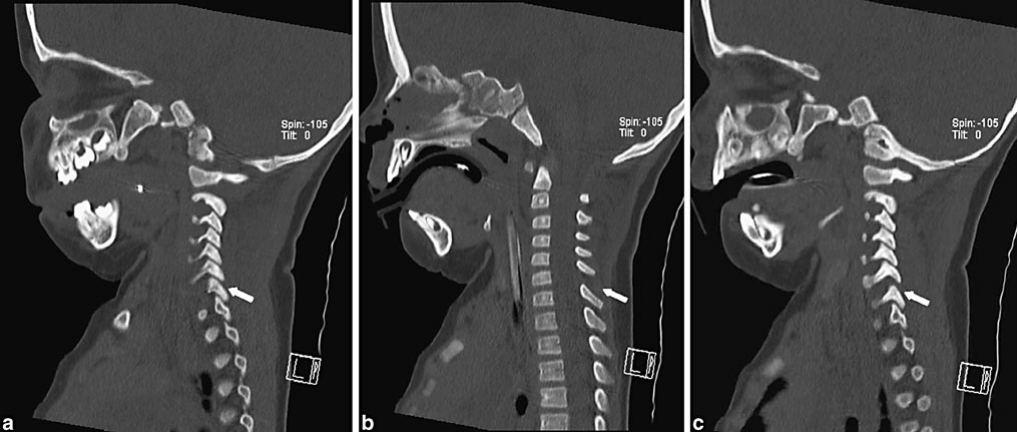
Sagittal reconstruction of the initial CT of the cervical spine. A normal alignment and distance is seen at facet joints between C6 and C7 *on the left* (a) as opposed to a diastasis and subluxed state of the facet joint at the same level *on the right* (c) The midline sagittal reconstruction (b) shows an increased distance between the interspinous processes of C6 and C7 indicating an injury to the interspinous ligament at that level. The alignment between C6 and C7 is still normal
